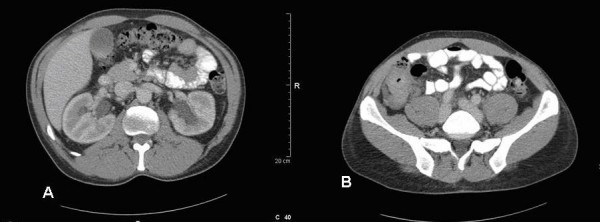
(a&b) – CT Scan: Bilateral hydronephrosis with associated caecal mass (arrow showing hydronephrosis).
